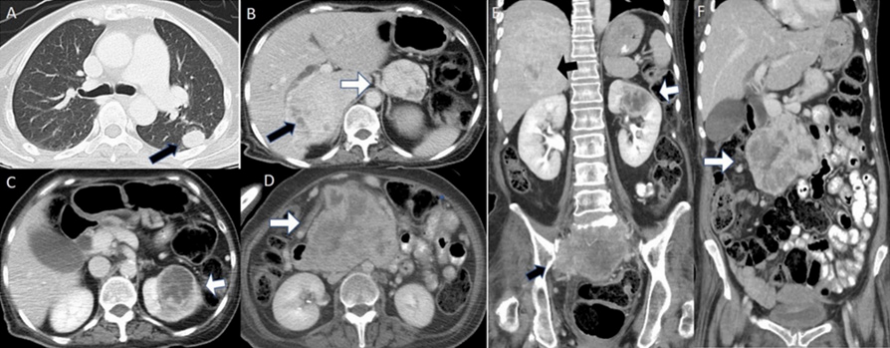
Follow-up CT thorax in the lung window (3 years later after defaulting treatment) shows enlargement of the nodular lesion in the apical segment left lower lobe (A, arrow). Follow-up axial and coronal contrast-enhanced CT scans of the abdomen in the portal venous phase show enlargement of heterogeneously enhancing lesions in hepatic segment VI/VII (B, E, black and white arrows), left kidney (C, E, white arrows) and pancreas head and uncinate process (B, D, F, white arrows). Also, note is made of the recurrent tumour in the sacrum (E, black arrow). These findings are consistent with tumour progression from metastatic spread of haemangiopericytoma.
